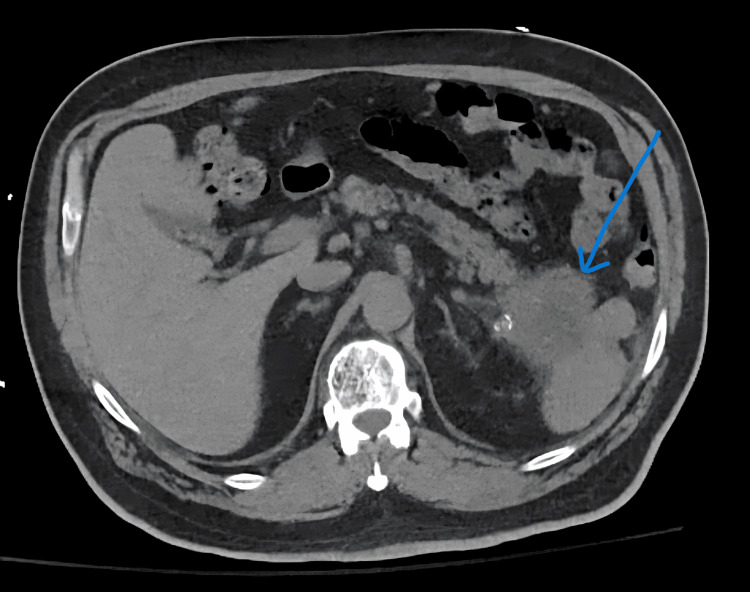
CT of the chest, abdomen, and pelvis showing a 5.7×5.5 cm mass arising from the pancreatic tail and invading the spleen CT: computed tomography
